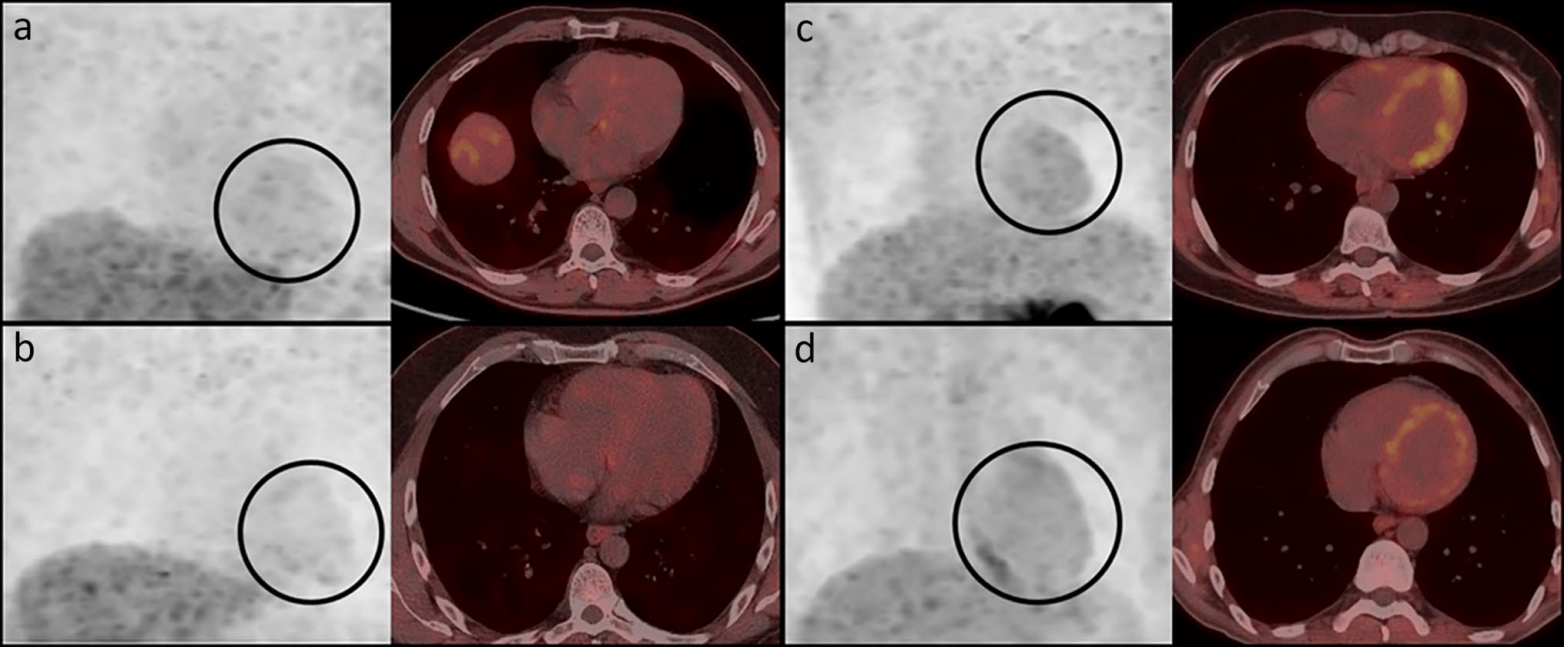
The maximum intensity projection (with the heart highlighted by a black circle) and trans-axial myocardial 18 F-DOPA PET/CT images of each patient are presented side by side. Decreased myocardial 18 F-DOPA uptake was observed in IPD patients with disease duration of 3 years (a) and 5 years (b), whereas relatively preserved myocardial 18 F-DOPA uptake was seen in AR-PD patients with 8 years (c) and 15 years (d) of duration
